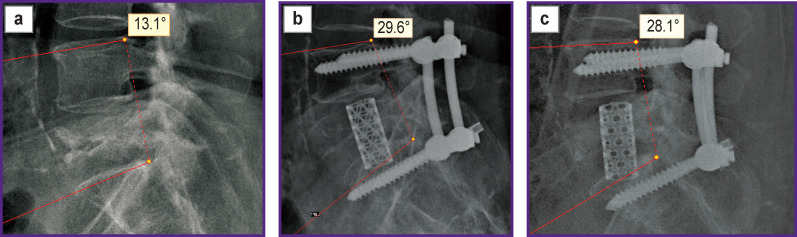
CT scan and X-ray examination of patient M., 53 years old: (a) 7 days after injury; (b) 7 days after transpedicular fixation and anterior column support with a Mesh; (c) 12 months after transpedicular fixation and anterior column support with a Mesh
